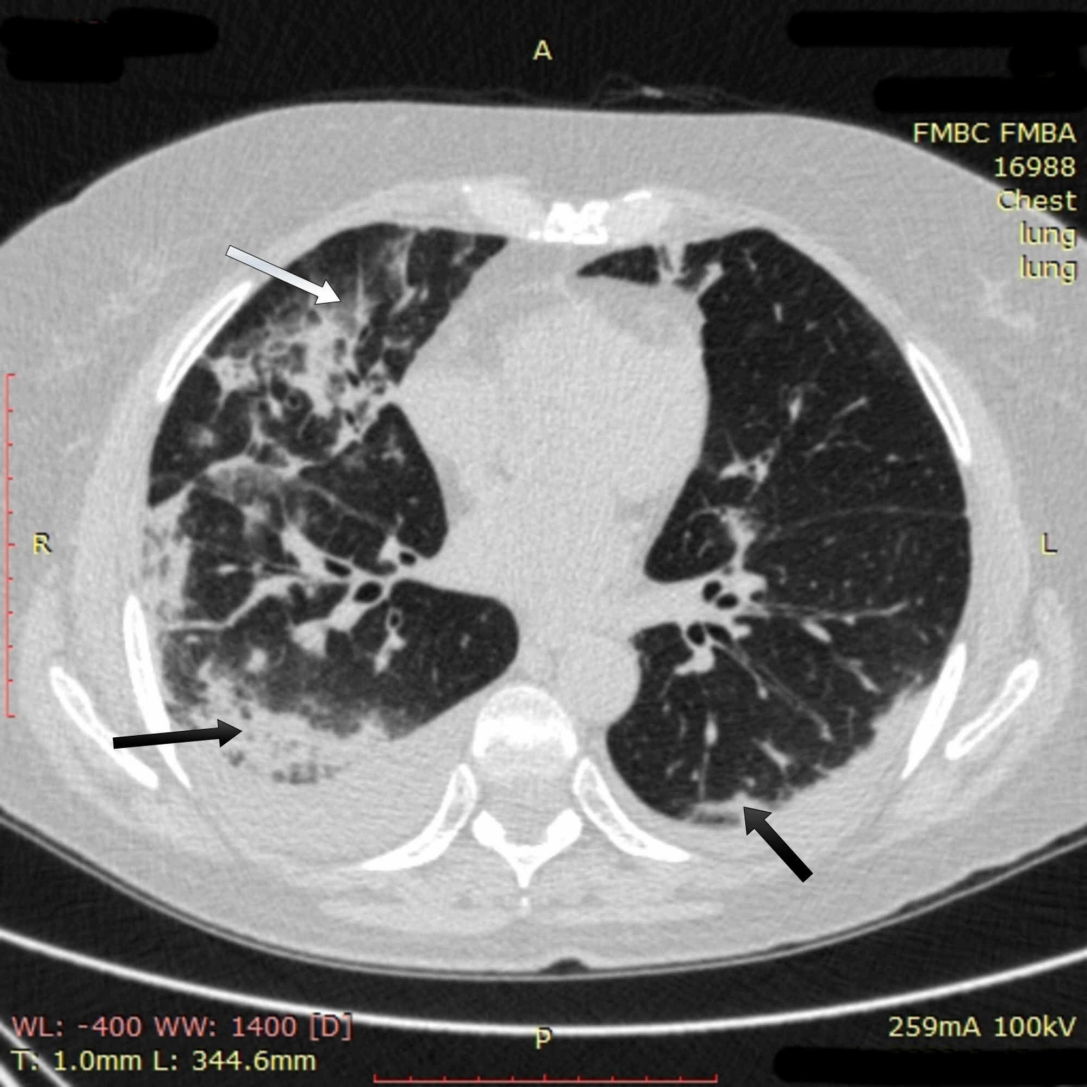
Chest CT imaging of patient 1 on day 11 Chest CT on day 11 showed multiple bilateral ground-glass opacities in subpleural and central regions of the middle and upper right lobes with a noticeable reticular pattern (white arrow); subpleural areas of consolidation in both lungs (black arrows) were also seen CT: computed tomography
